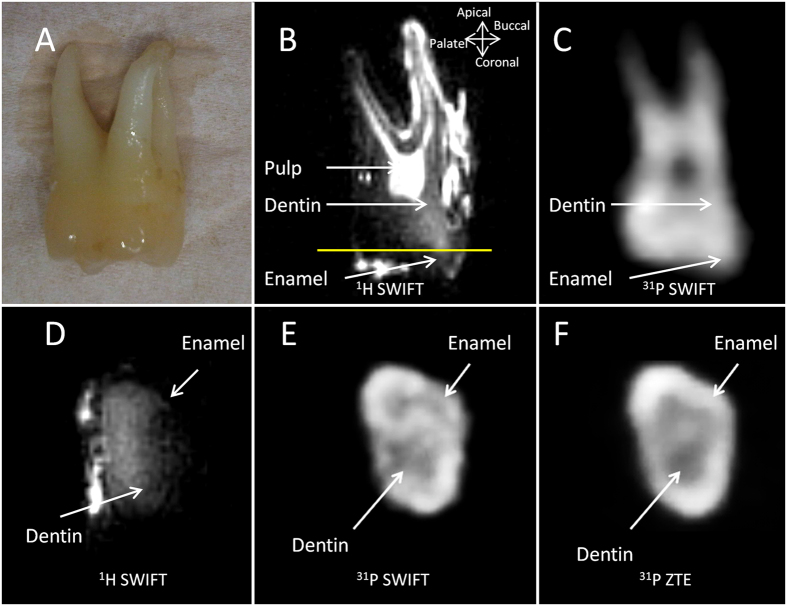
Photograph (A) and selected slices of 3D MR images of human maxillary left second molar tooth (B–F) including SWIFT ^1^H images (B,D), SWIFT ^31^P images (C,E) and ZTE ^31^P image (F) presenting axial (B,C) and apical (D–F) slices. The position of apical slices (D–F) is represented by the yellow line in image B. In the images of this slice, dentin and enamel are clearly separated. Image acquisition times were 100 s (B,D), 60 s (C) and 14  min (E,F).
